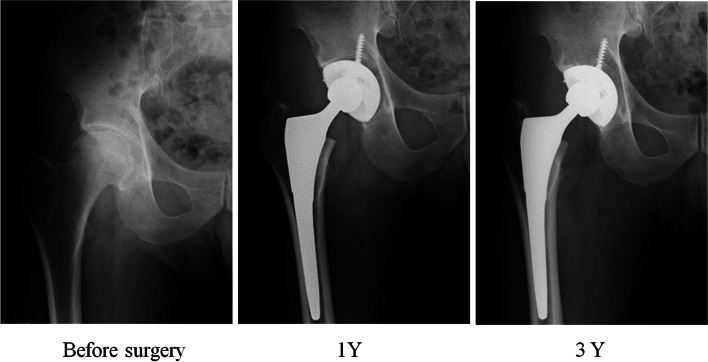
Radiographs of the representative case without stress shielding. A Before surgery. B 1 year after surgery. C 3 year after surgery
